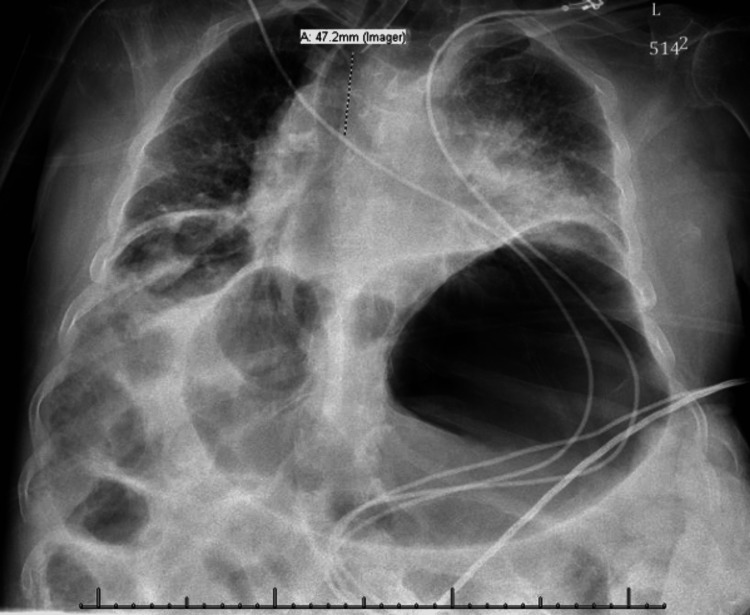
Chest X-ray showing large gastric bubble pre-OG tube placement OG: orogastric
